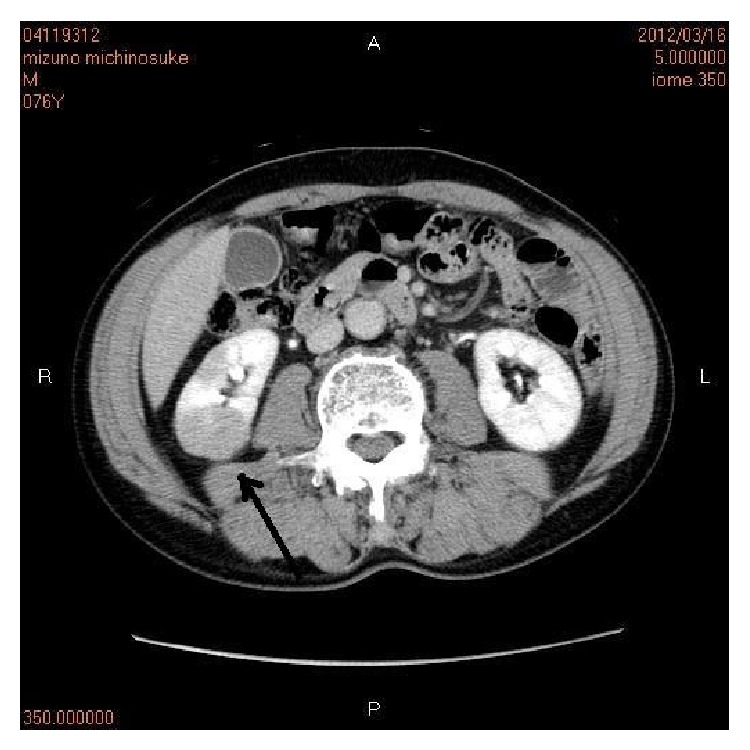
Enhanced CT findings. The right kidney (arrow) shows irregular enhancement compared to the left kidney. The findings suggest renal pelvic carcinoma.
